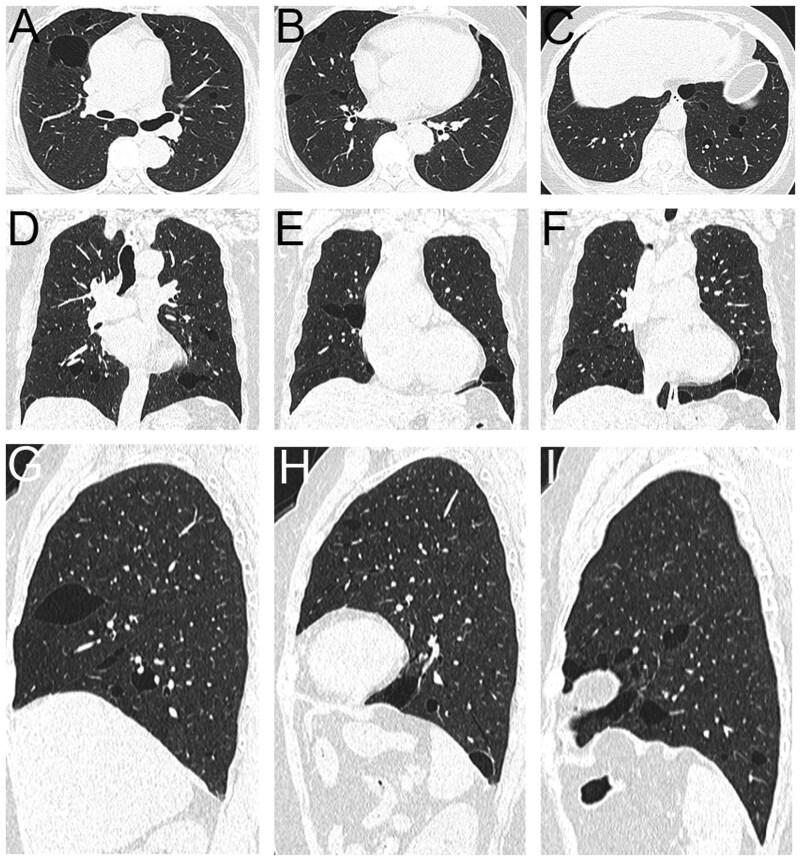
FDS in a 58-year-old woman. CT images (A-C, axial; D-F, coronal; G-H, sagittal) show multiple, well-defined, thin-walled pulmonary cysts of varying size and morphology—round, oval, and irregular—clustered in the medial, mid-lung, and lower lobes, with a characteristic subpleural distribution along the mediastinal pleura, interlobar fissures, and costophrenic angles. Abbreviation: FDS = folliculin deficiency syndrome.
